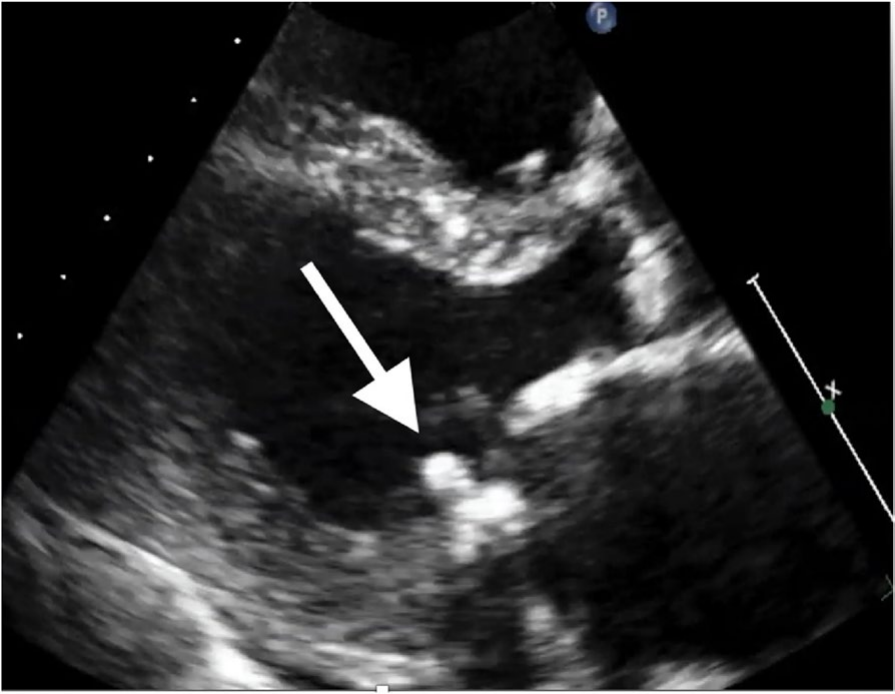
Echocardiography performed 2 months later. Transthoracic echocardiography shows a highly mobile mass (8 × 5 mm) attached to the mitral annulus (white arrow)
